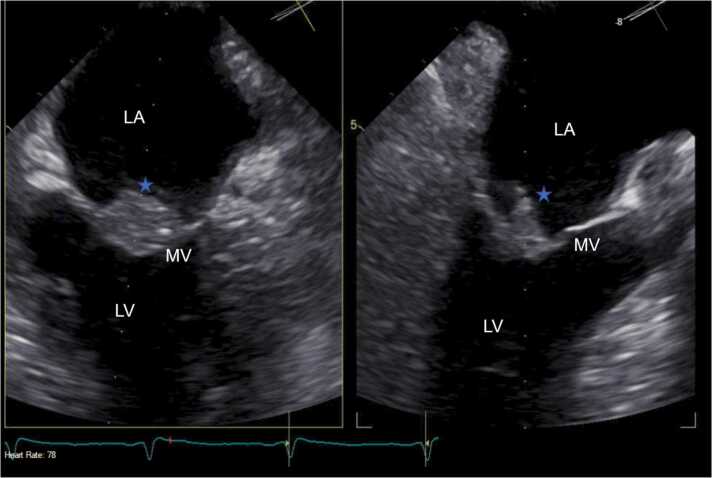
Transesophageal (TEE) Mitral commissural view revealing a large (1.73 × 0.55 cm), mobile vegetation (Blue star) on the atrial aspect of the anterior leaflet of mitral valve. LA = Left Atrium, LV = Left Ventricle, MV = Mitral Valve.
